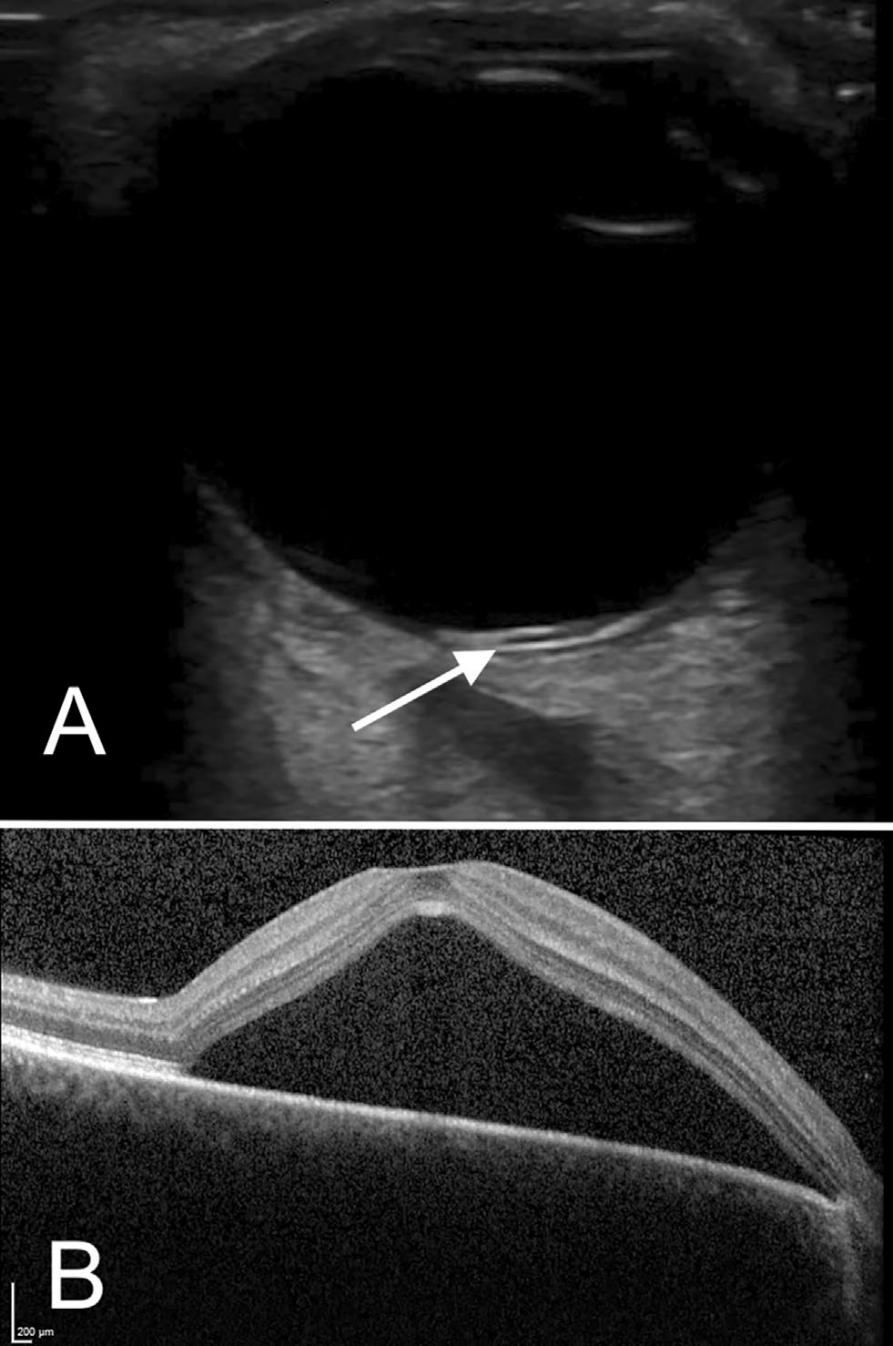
(A) High frequency transverse ultrasonography of the right eye with a linear probe. The RPE is denoted by the white arrow. Serous retinal detachment is seen as an immobile, dome‐shaped non‐echogenic region anterior to the RPE, with a corresponding band of non‐echogenicity posteriorly, potentially representing choroidal hyper‐permeability. (B) Optical coherence tomography of the right eye demonstrates a large sub‐foveal serous retinal detachment extending to involve the superior macula.
